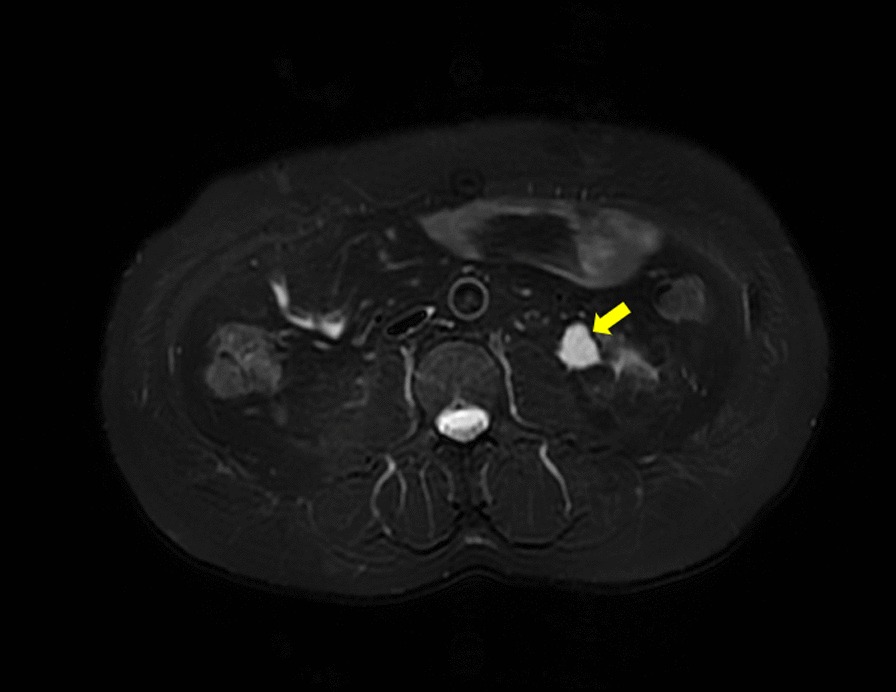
MRI appearances of the tumor demonstrated hyperintensity on T2-weighted images
